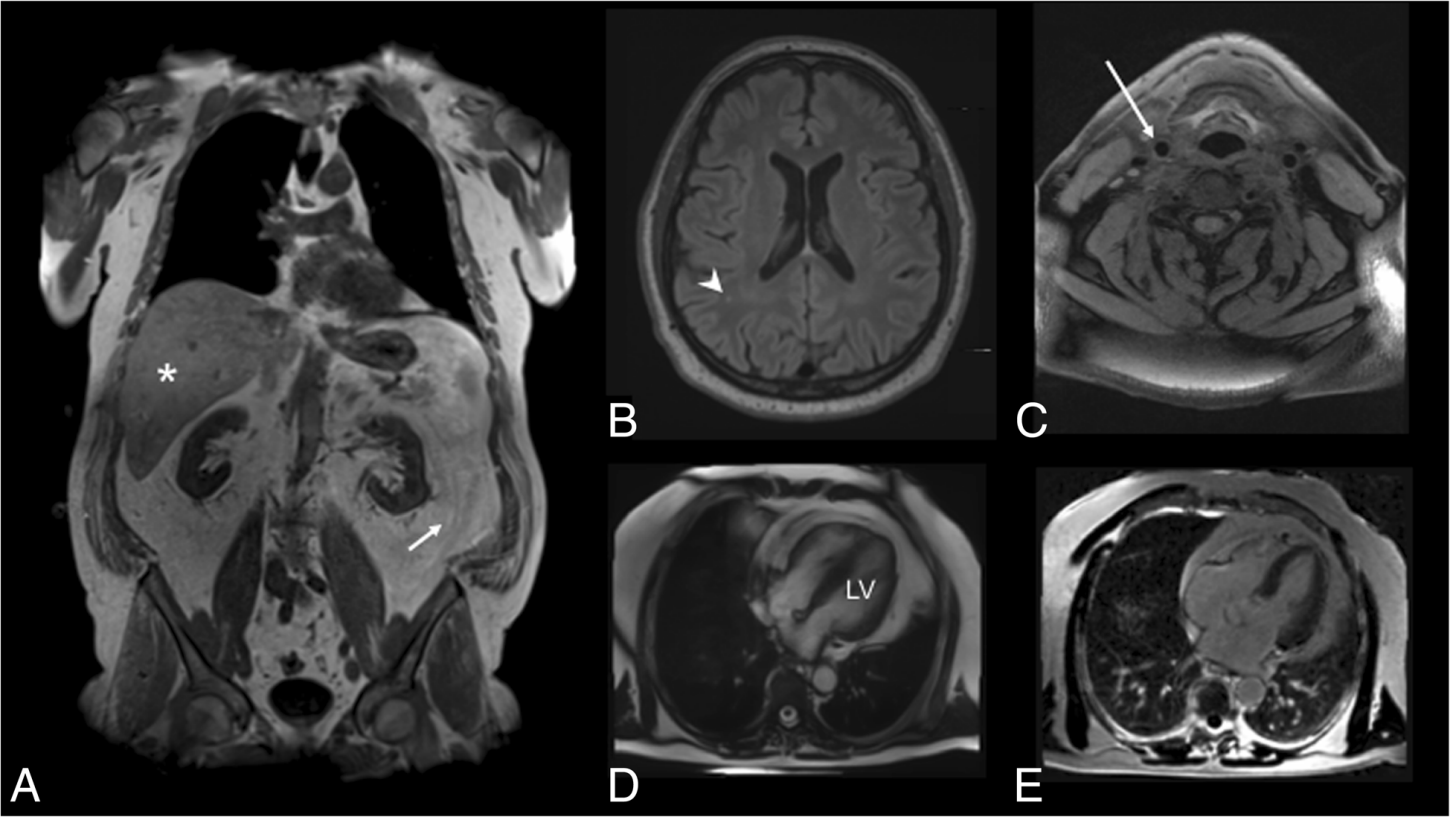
Imaging findings in a 61-year-old male indicating extensive subclinical disease burden. a Two-point DIXON T1-weighted sequence for the assessment of visceral adipose tissue volume from the femoral head to the cardiac apex (arrow) indicating high levels of fat as well as hepatic proton density fat fraction (asterisk, measured on multi-echo VIBE T1-weighted sequences). b Fluid-attenuated inversion recovery sequences demonstrating mild white matter lesions (arrowhead). c Atherosclerotic carotid plaque was determined on black-blood T1-weighted fat-suppressed sequences in the common carotid artery (arrow), the carotid bulb and the proximal internal carotid artery. d Cine-SSFP sequences were evaluated for the calculation of volume and mass left ventricle (LV). e late gadolinium enhancement was detected on fast-low-single-shot inversion recovery sequence four-chamber view [23]
